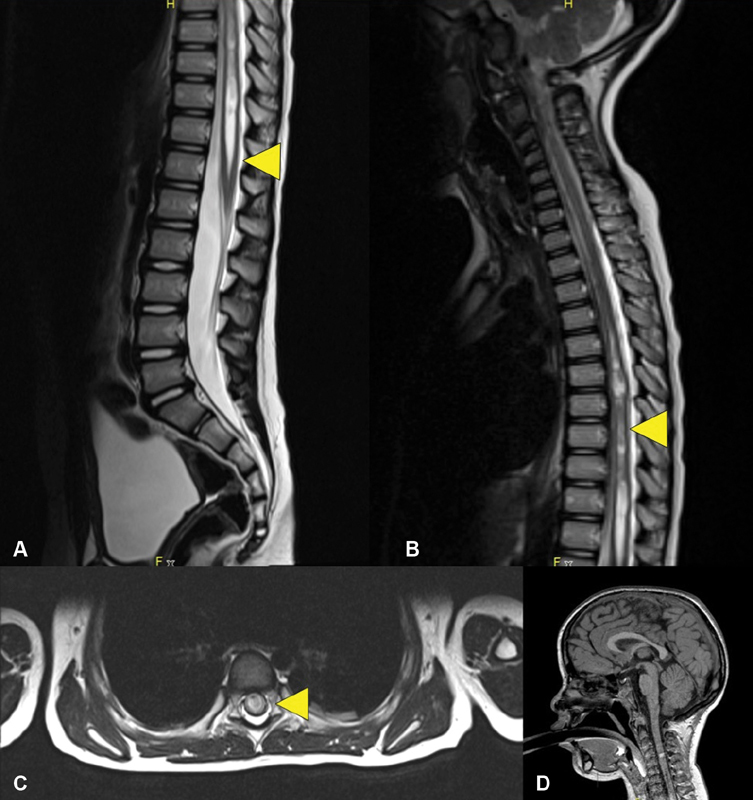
Sagittal T2-weighted magnetic resonance imagings (MRIs) of the patient showing syringohydromyelia from conus medullaris (
